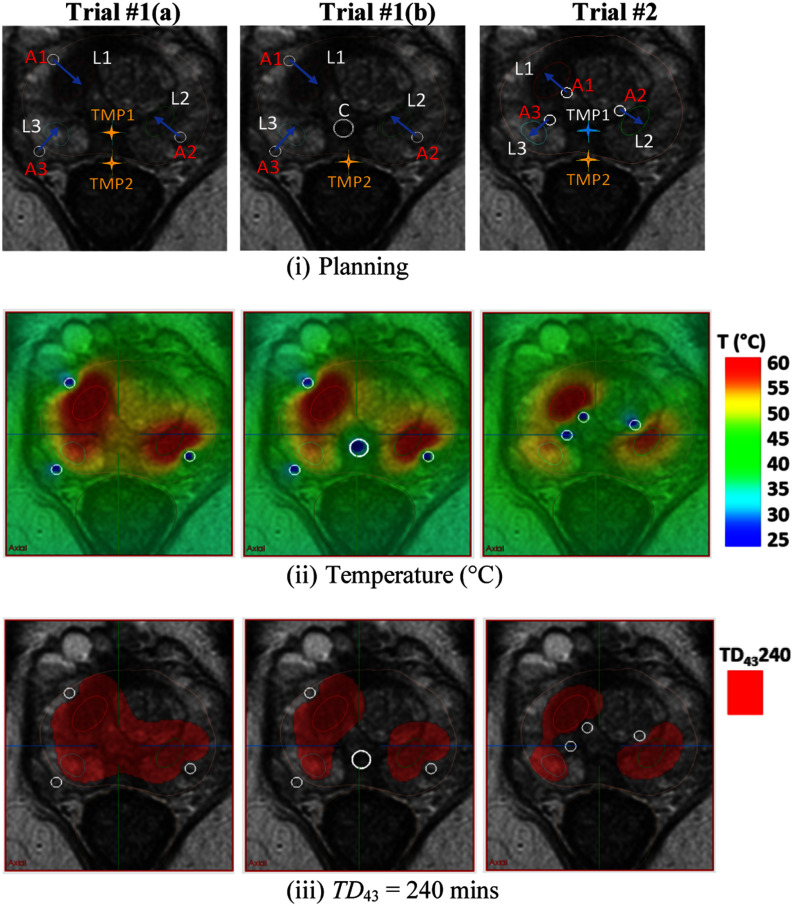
Comparison of ablation configurations including urethral cooling for patient case 12: Peripheral implant for Trial#1(a) A1-A3 directing inward; Peripheral implant for Trial#1(b) with A1-A3 directing inward with integrated urethral cooling; and central implant for Trial #2 with A1-A3 directing outward. Shown are (i) thermal treatment planning based on MP-MRI, (ii) temperature (*T*, °C), and (iii) lethal thermal dose (*TD_43_* 240, mins) distributions, shown as red overlay.
